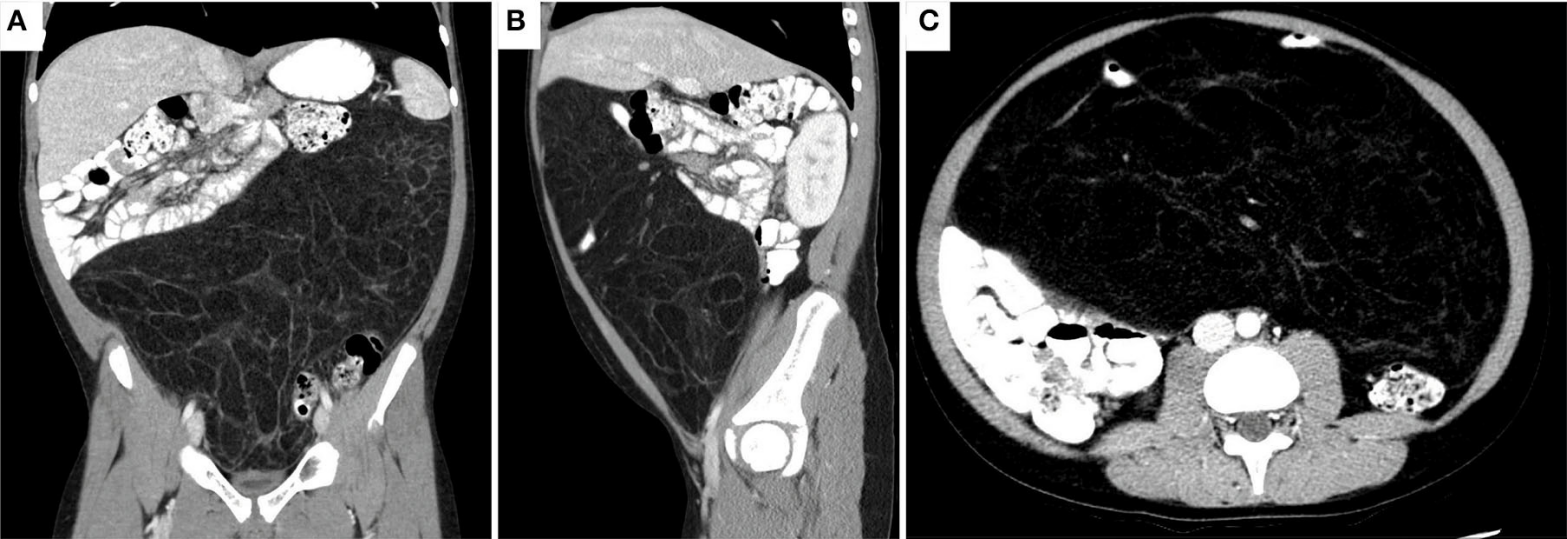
CT abdomen/pelvis revealing a 33 x 27 x 15 cm, predominant fatty mass with multiple septa and a few areas of soft tissue density. The mass displaces most of the abdominal contents posteriorly. A loop of small bowel is seen within thin the mass. No evidence of obstruction noted as oral contrast reached the sigmoid colon. (A) Coronal (B) Sagittal (C) Axial views.
